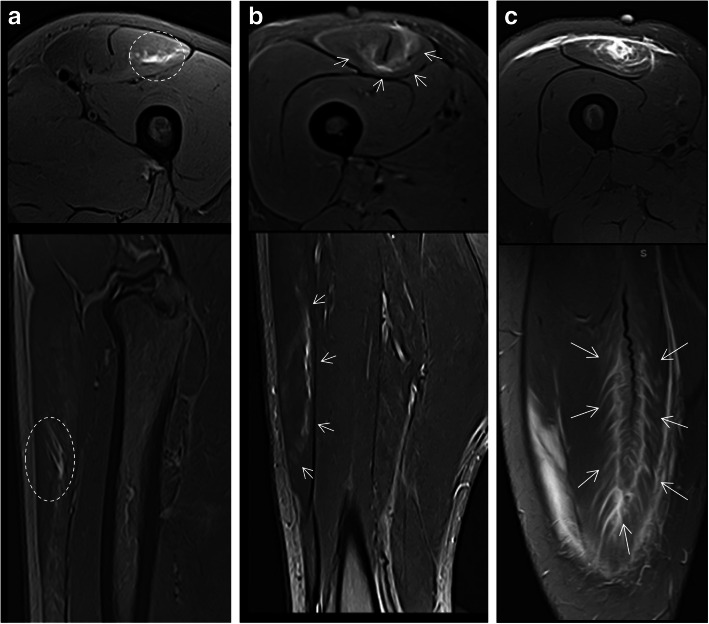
Intramuscular isolated injuries of the rectus femoris. Axial and coronal/sagittal MR images in fluid-sensitive sequences. a Marginal or paraseptal muscular edema with gap of the muscle fibers (circles). b Edema, fiber distortion, and areas of tear surrounding the central septum (short arrows) without retraction. c Circumferential pattern of fibers rupture encompasses the entire central septum with loss of its tension (large arrows)
